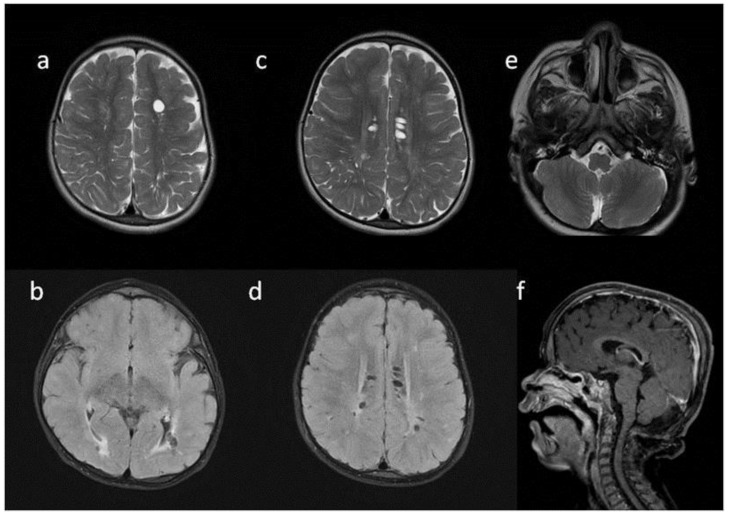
Brain MRI. Patient 2. (a,c,e) axial T2; (b,d) axial T2 FLAIR; (f) sagittal T1. Findings: (a,c,d) enlarged PVS with characteristic location in corpus callosum; (a–d) abnormal white matter signals; (e) posterior fossa horns; (f) J-shaped sella turcica, dysplastic dens.
